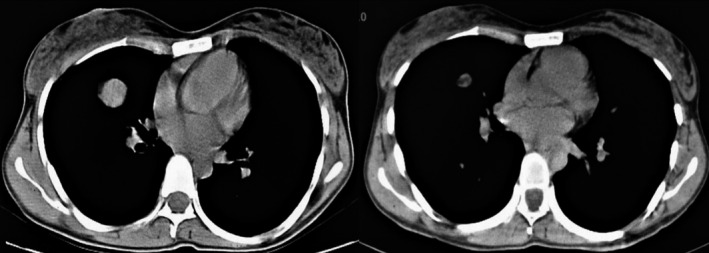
Axial CT scan views showing pulmonary metastasis before(33 × 28 mm) and 6 mo after (13 × 9 mm) chemotherapy with gemcitabine + docetaxel
